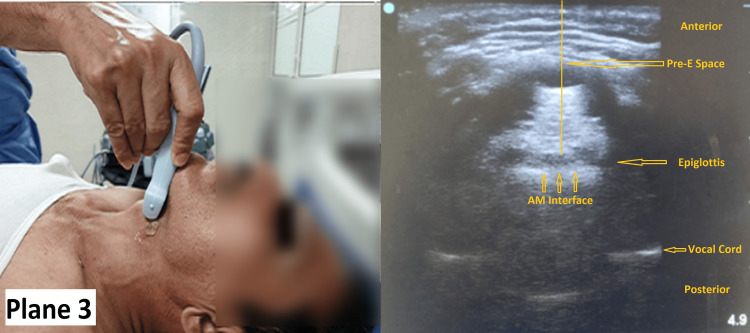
Measurements of Pre-E and E-VC Note the position of the ultrasound probe on the neck (Plane 3) and the corresponding image Pre-E: pre-epiglottic space, E-VC: epiglottis to vocal cord distance, AM: air mucosal
